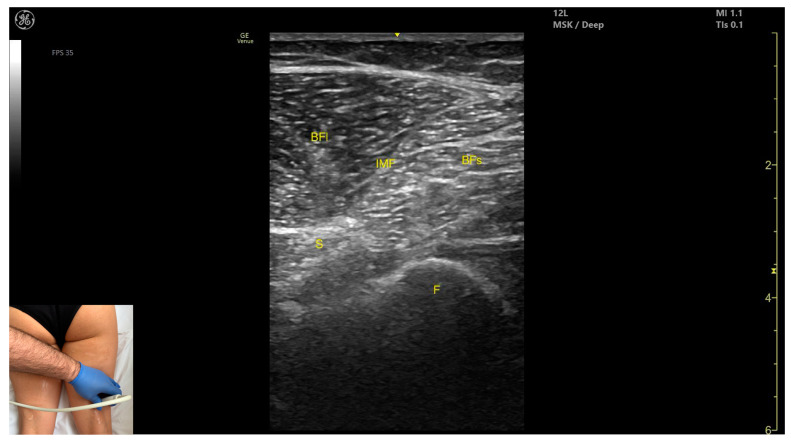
Ultrasound anatomy of *biceps femoris* (BF) with key landmarks: BFl—*biceps femoris* long head; IMF—intramuscular fascia; BFs—*biceps femoris* short head; S—sciatic nerve; F—femur.
